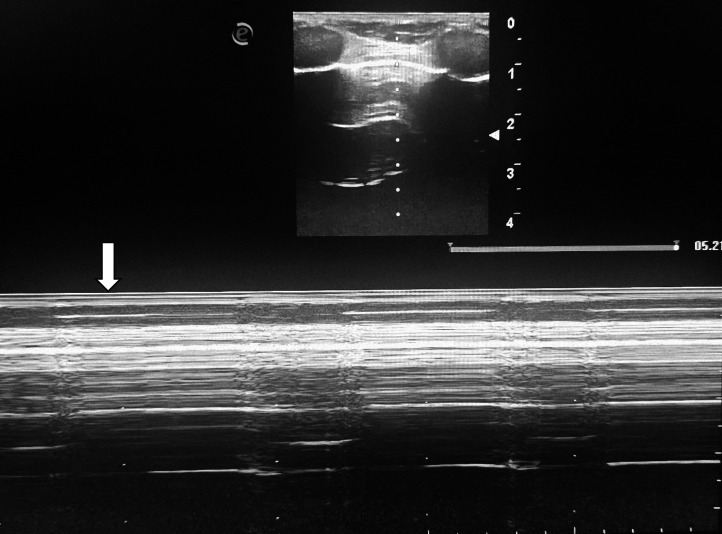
M-mode lung ultrasound showing the barcode sign (absence of pleural sliding), consistent with pneumothorax (with arrow).
